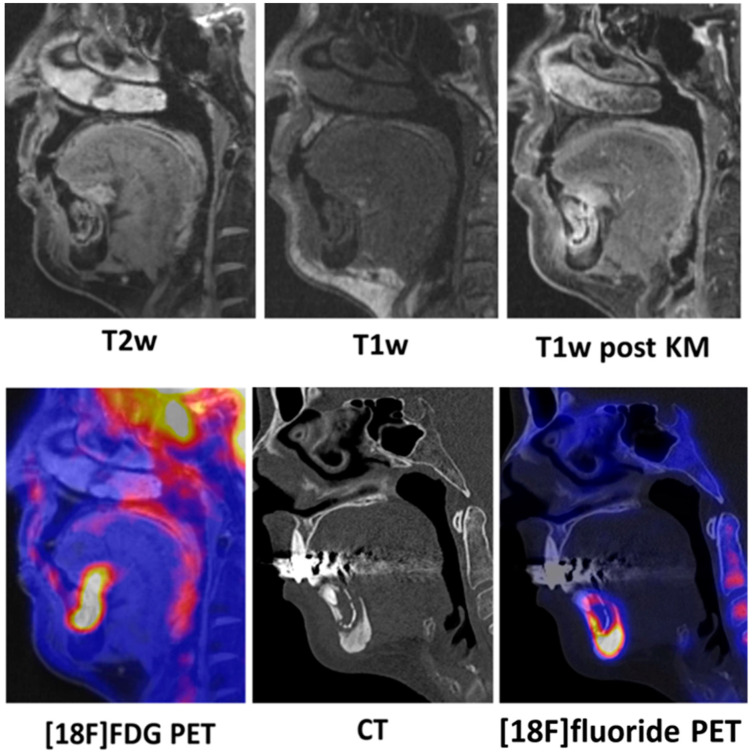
A 58-year-old female patient diagnosed with medication-related osteonecrosis of the jaw (MRONJ) in the mandible. Sequestrations are visible in regions 34 to 43. In the sagittally reconstructed images, the sequestration area within the jawbone appears hyperintense in T2-weighted MRI images and hypointense in T1-weighted images, indicating bone edema. In the post-contrast T1-weighted images, there is a notable enhancement of gadolinium in the affected mandible and, to a lesser extent, in the surrounding soft tissue. To a comparable extent, there is an uptake of [18F]fluorodeoxyglucose (FDG) in the sequestration area and adjacent soft tissue, consistent with inflammation-related granulation tissue. Moreover, no significant soft tissue inflammation was observed. On CT imaging, there is evidence of increased sclerosis in the mandible surrounding the sequestration area, accompanied by corresponding elevated [18F]fluoride uptake on positron emission tomography (PET), while the sequestration area itself shows no [18F]fluoride uptake. Overall, the extent of regions with increased metabolic activity on [18F]fluoride PET was smaller than the morphological changes detected by CT and MRI.
